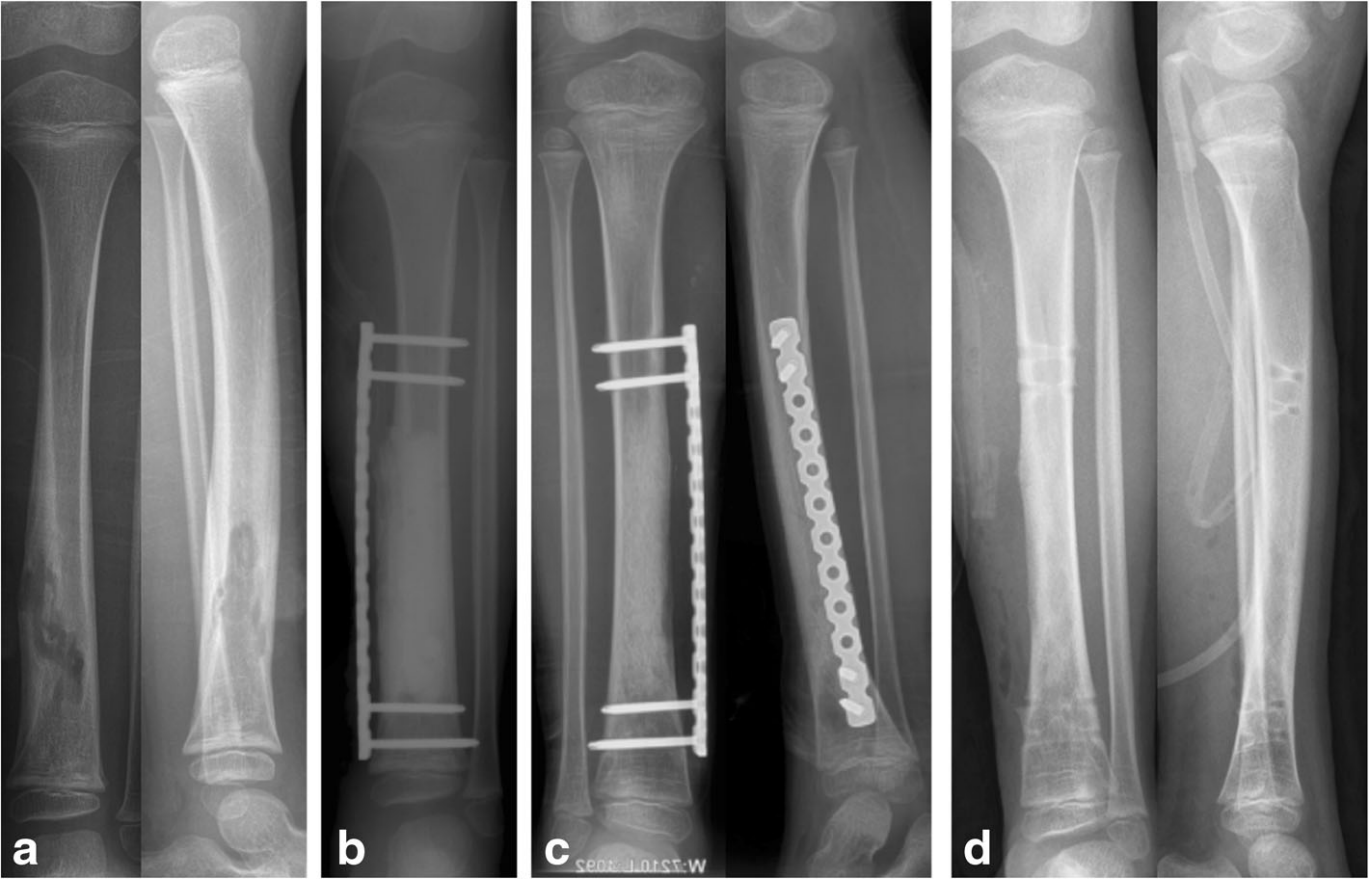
Case 8, 6 Y, Redness and painful left tibia for 3 M. The patient had a history of a fall 2 weeks before the illness; however, fracture and skin breakdown were not noted. Bone reconstruction was performed with an allograft. a: X-ray revealed bone destruction of the distal tibia; b: Implanted with PMMA cement and fixed with plate after debridement; c: X-ray revealed bone union 3 months after grafting; d: The bone defects were completely healed, and fixation was removed after 18 months
